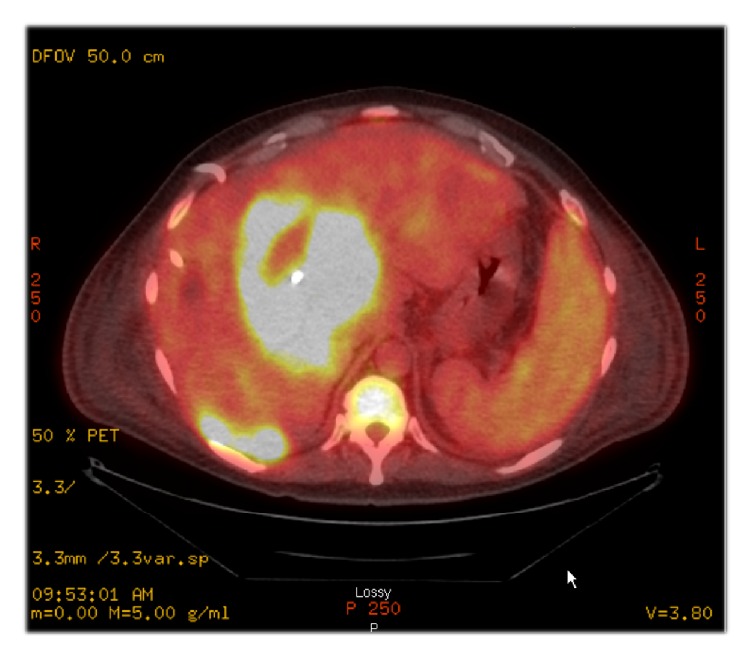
Postbiopsy PET/CT result.
